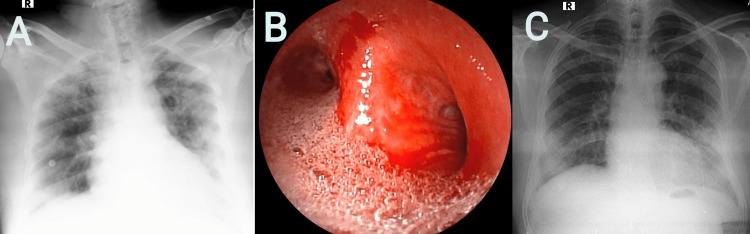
A) Chest radiograph revealed an enlarged cardiac silhouette with bilateral diffuse infiltrates. B) Flexible bronchoscopy revealed frothy secretions flooding the bilateral mainstem bronchi. C) Resolution of pulmonary edema in chest radiograph.
